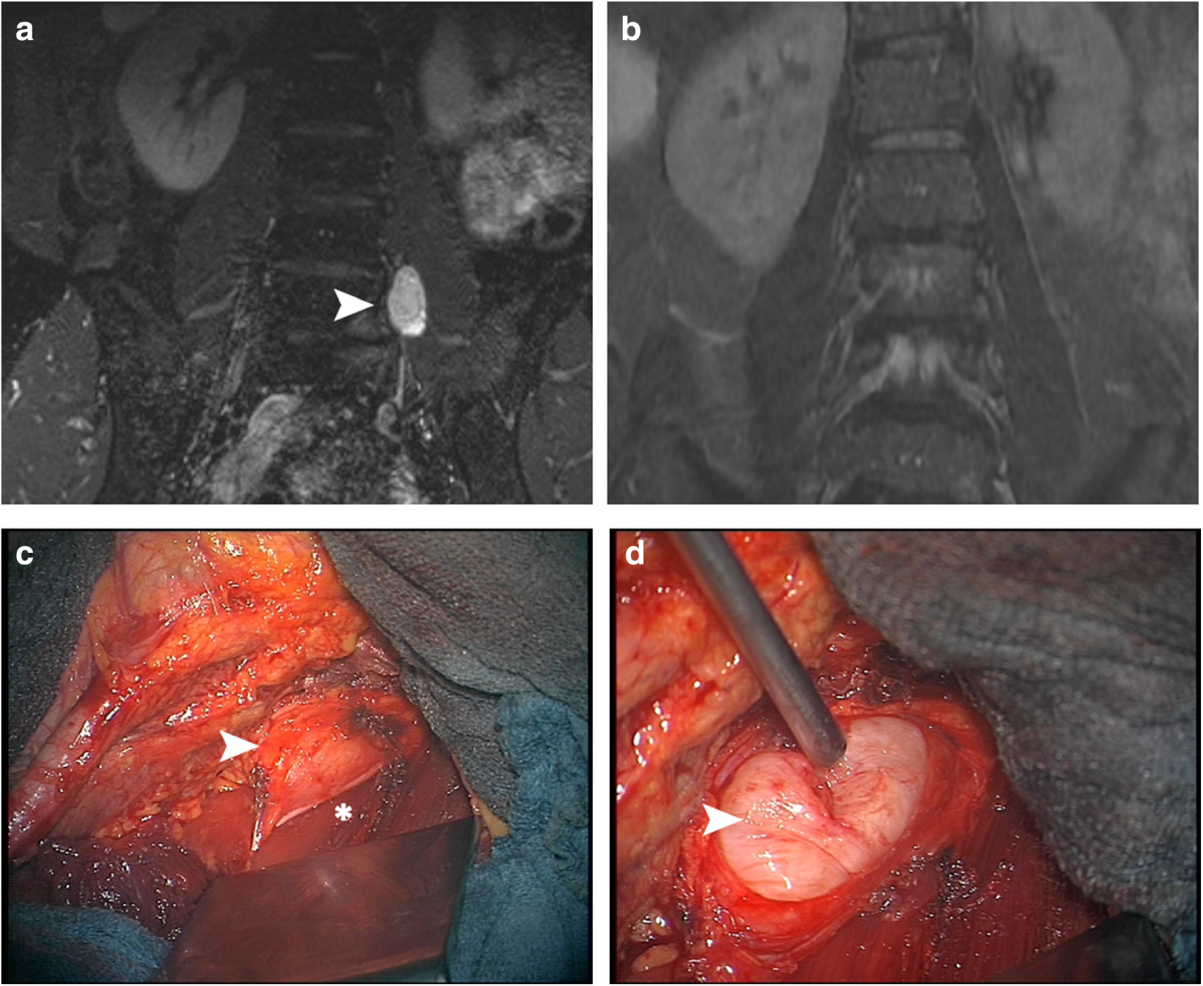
Pre- (A) and postoperative (B) MRI of a retroperitoneal PNST (white arrow). Once the general surgeon had exposed the tumor behind the psoas muscle (*), the neurosurgeon incised the pseudocapsule (white arrow in C), dissected the plane between the pseudocapsule and the true tumor capsule and removed the tumor (white arrow in D)
